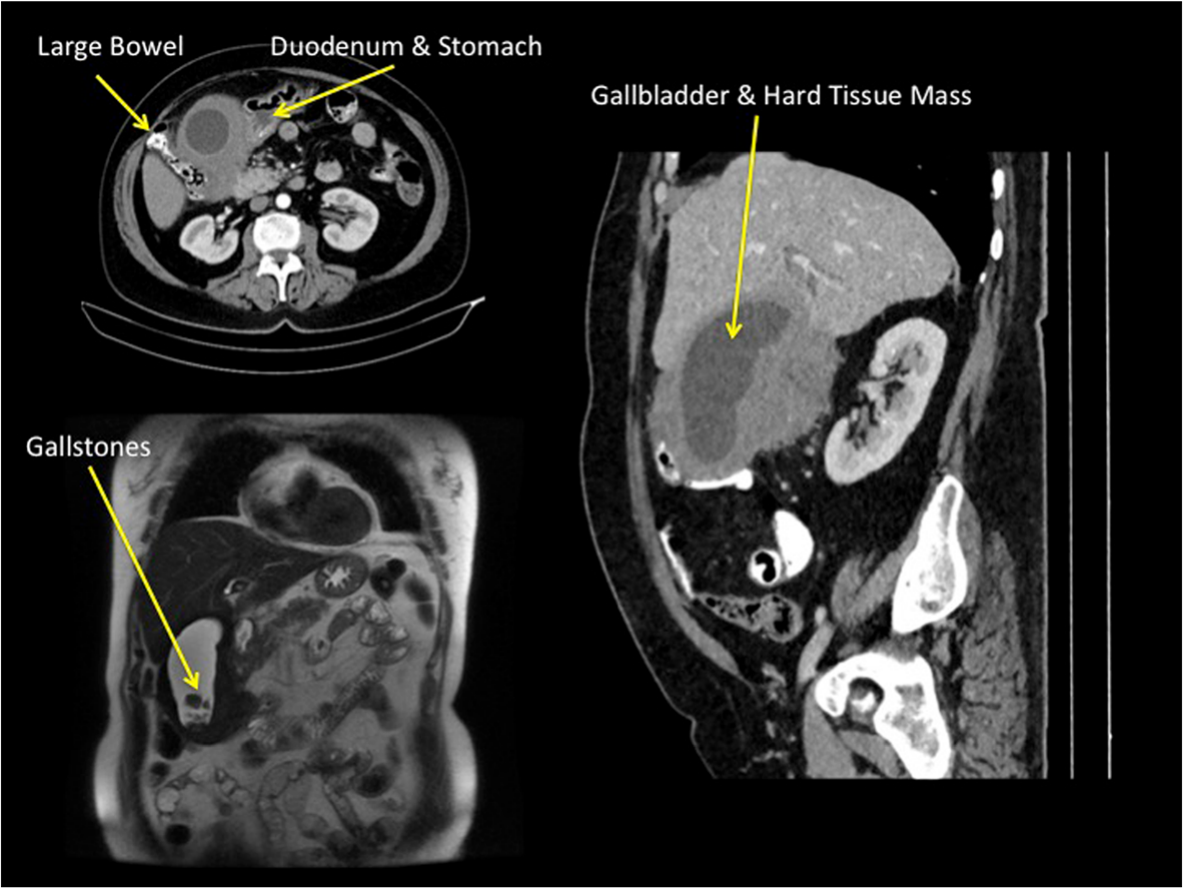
Axial and coronal computed tomography images showing a large mass of diffuse soft tissues originating from the gallbladder, and displacing the duodenum, transverse colon and hepatic flexure (a, b). In magnetic resonance imaging the gallbladder was distended and contained stones, associated with a slight dilatation of the intrahepatic bile duct (c)
